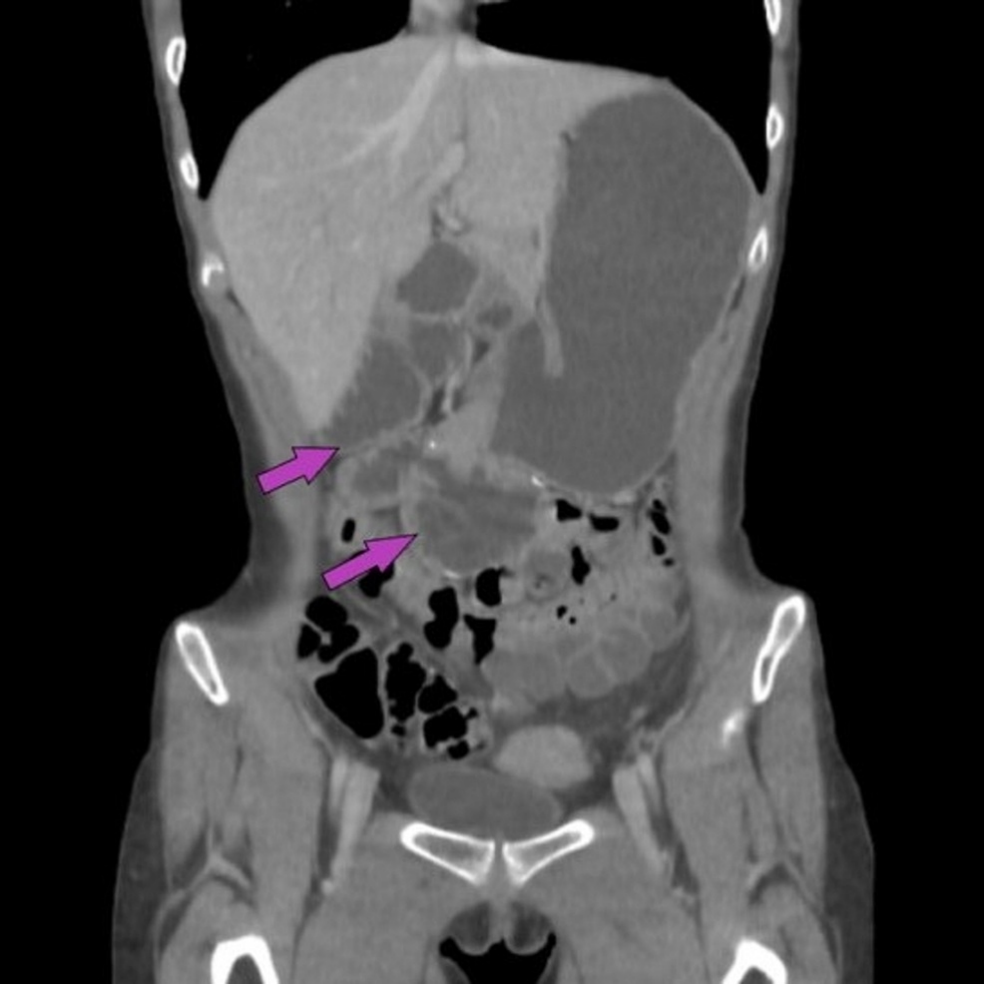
CT enterography demonstrating persistent dilation of the stomach and proximal duodenum six years post-operatively Arrows indicate dilated loops of the proximal duodenum.
